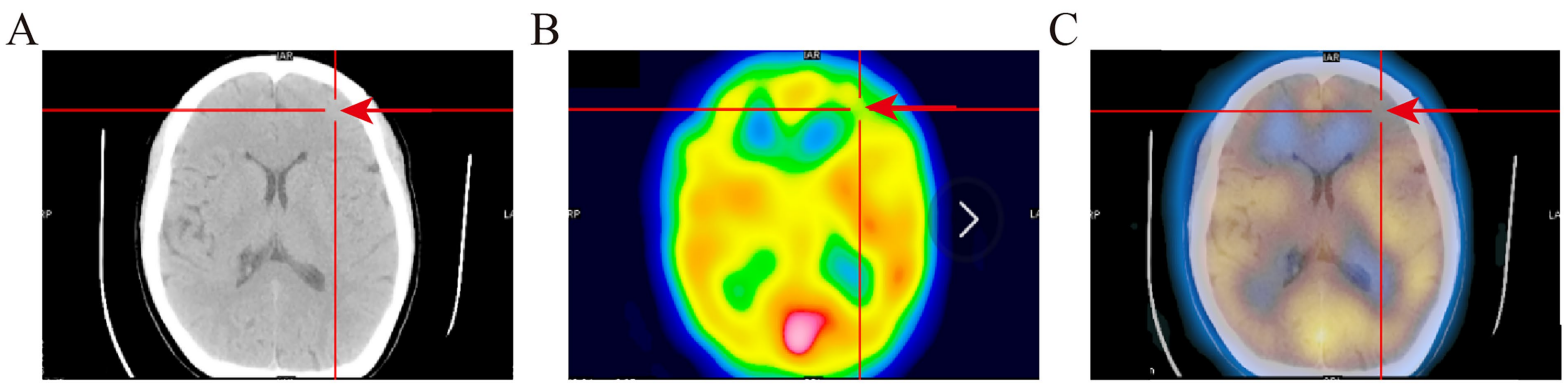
The SPECT/CT images of PWE. (A) The CT image. (B) The SPECT image. (C) The fusion image; Figure shows that the left frontal lobe has hypoperfusion than the contralateral side. Red arrow indicates ischemic lesion.
